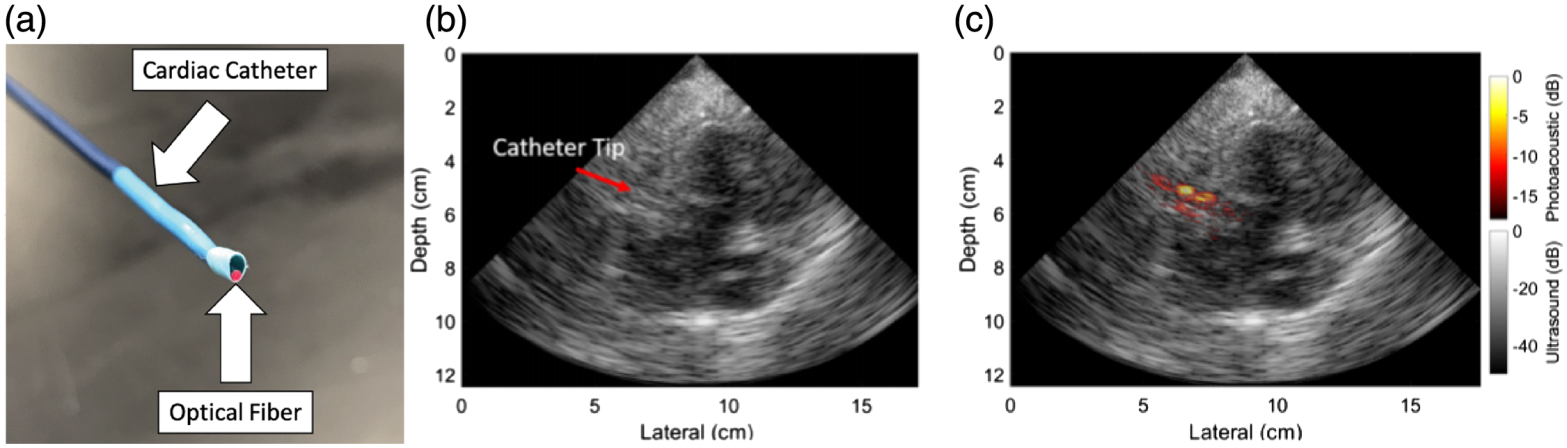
(a) A 1 mm-core-diameter optical fiber was inserted with its tip coincident with the distal tip of a 5F inner diameter, 7F outer diameter cardiac catheter. (b) Ultrasound image and (c) PA image overlaid on the corresponding ultrasound image, each showing the catheter tip in contact with the right ventricular outflow tract. The ultrasound and PA images were acquired with a subcostal acoustic window and provided depth information that is not present in the fluoroscopic images. The catheter tip location and its contact with the endocardium are more apparent in the PA image when compared to the ultrasound image. Adapted from Ref. 222, available under a CC-BY 4.0 license.
